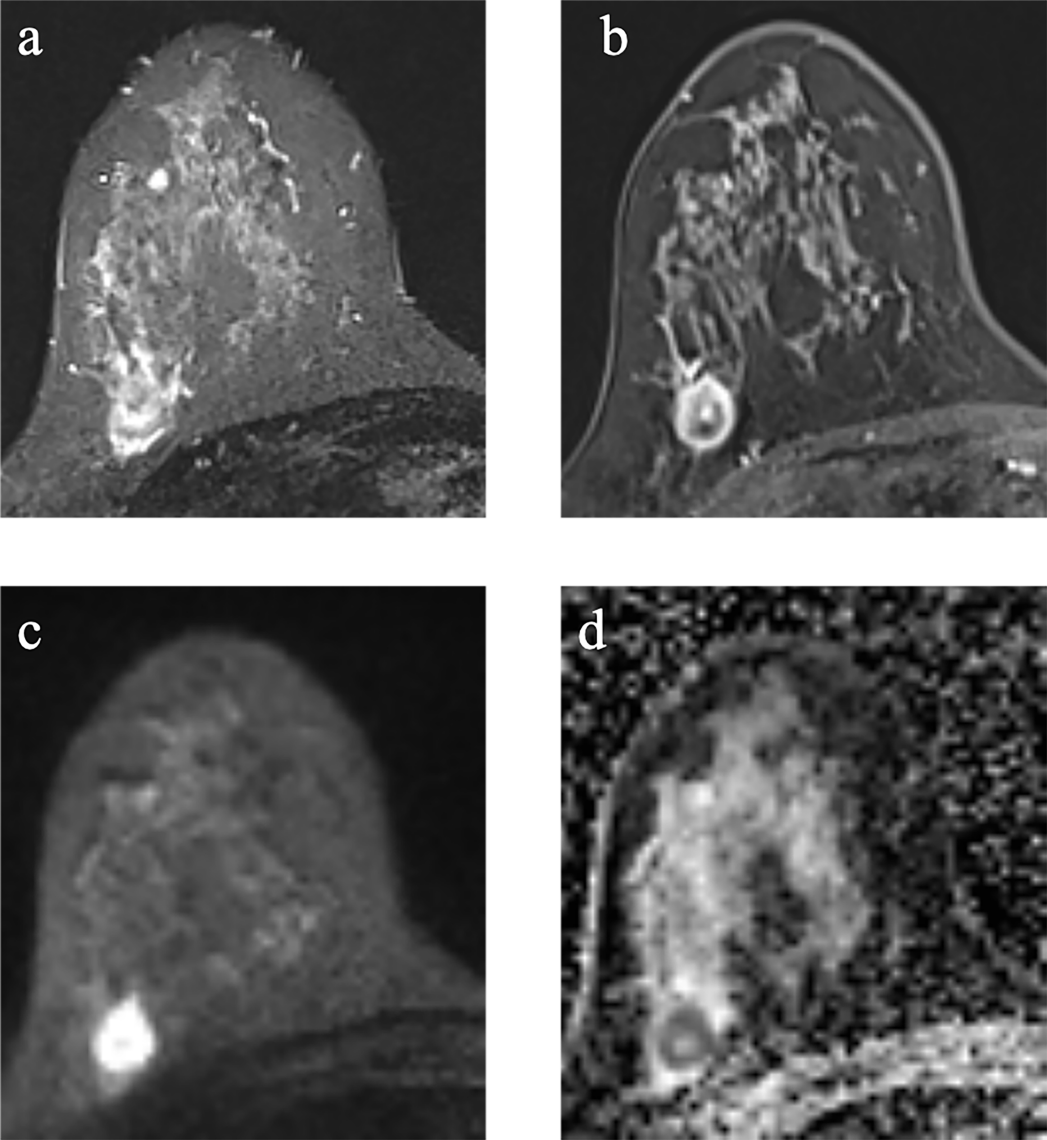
Example images of a 29-year-old woman with TNBC with TP53 mutation in the right breast (A-D). (A): T2-weighted-image. (B): the first phase of T1WI after contrast enhancement. (C): diffusion-weighted image of b_1000_. (D): apparent diffusion coefficient (ADC) map.
